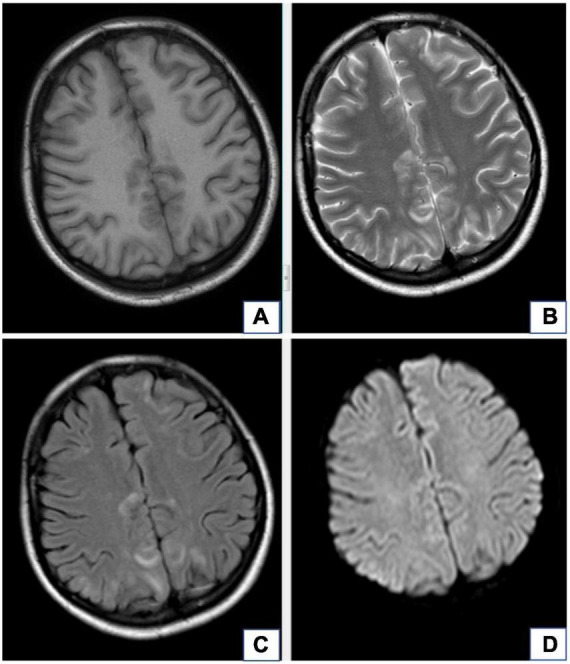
A 20-year-old female with diagnosis of preeclampsia-related reversible posterior leukoencephalopathy syndrome. The lesions occur in bilateral parieto-occipital lobes, which are isointense or hypointense on T1WI images (A), hyperintense on T2WI images (B), and hyperintense on T2-FLAIR (C), but there is no obvious hyperintense signal in these lesions on DWI (D).
